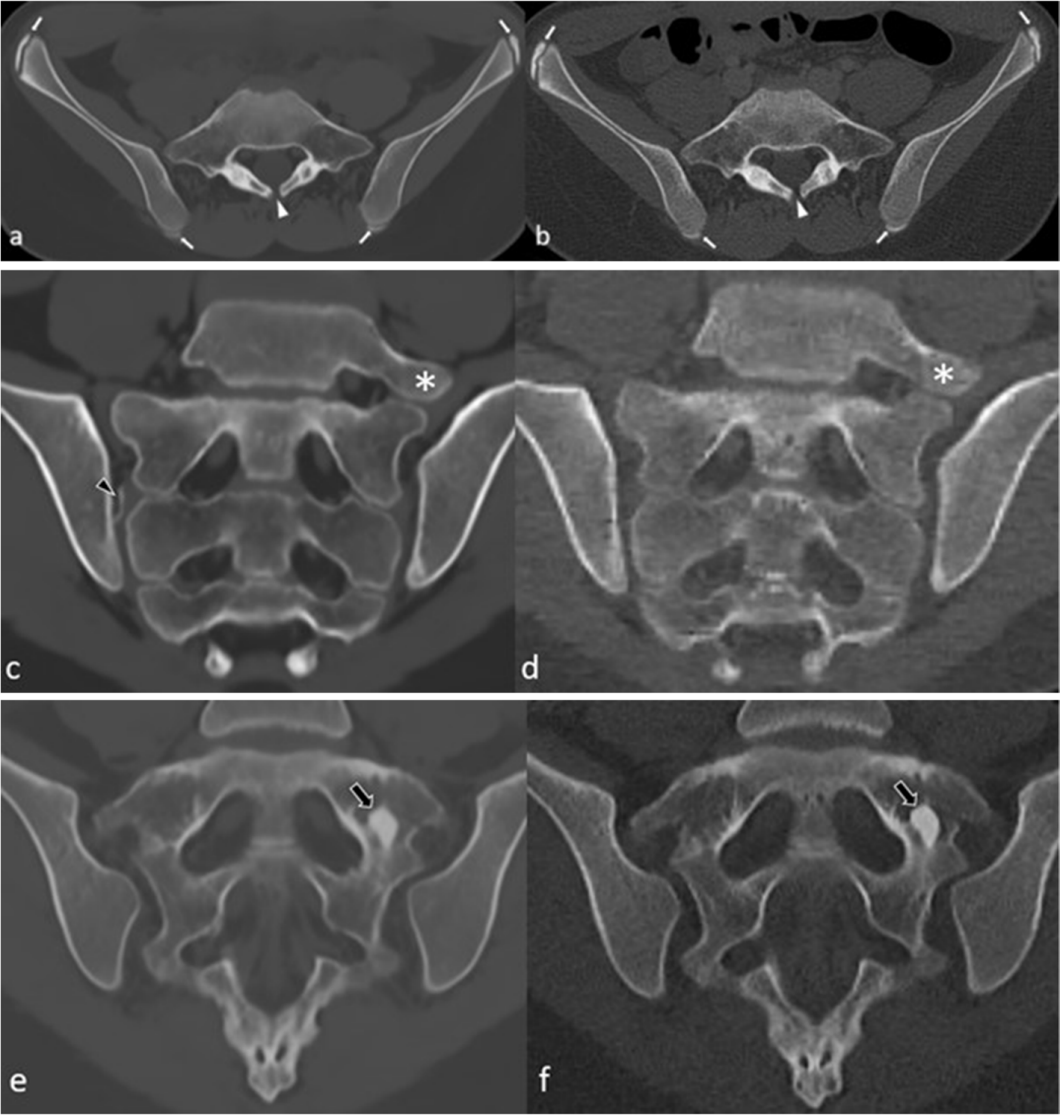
Some incidental findings were seen on both MRI-based synthetic CT (sCT) (left images a, c, and e) and CT (right images b, d, and f). a–b Spina bifida occulta of S1 (arrowhead) in a 15-year-old girl in an axial plane. Also note the ossification centers of the apophysis of the iliac crest that can be seen as well (arrows). c–d Lumbosacral transitional anomaly without bony fusion on the left side (asterisk) in a 12-year-old boy in a paracoronal plane. Also note the white line in the right SI joint (black arrowhead), consistent with an artifact on sCT (this was not seen on CT). e–f Enostoma in a 16-year-old female on the left side of S1 in a paracoronal plane (black arrows)
